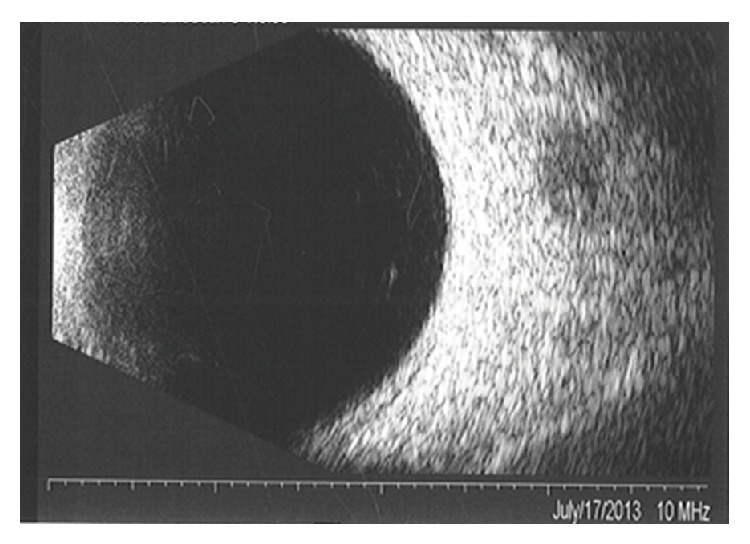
B-scan ultrasound showed a complete regression of the choroidal lesion after 4 cycles of chemotherapy and intravenous bevacizumab.
